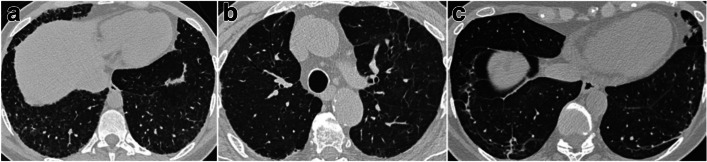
HRCT images showing diferent fibrosis patterns. a Honeycomb. b Traction bronchiectasis. c Architectural distorsions
